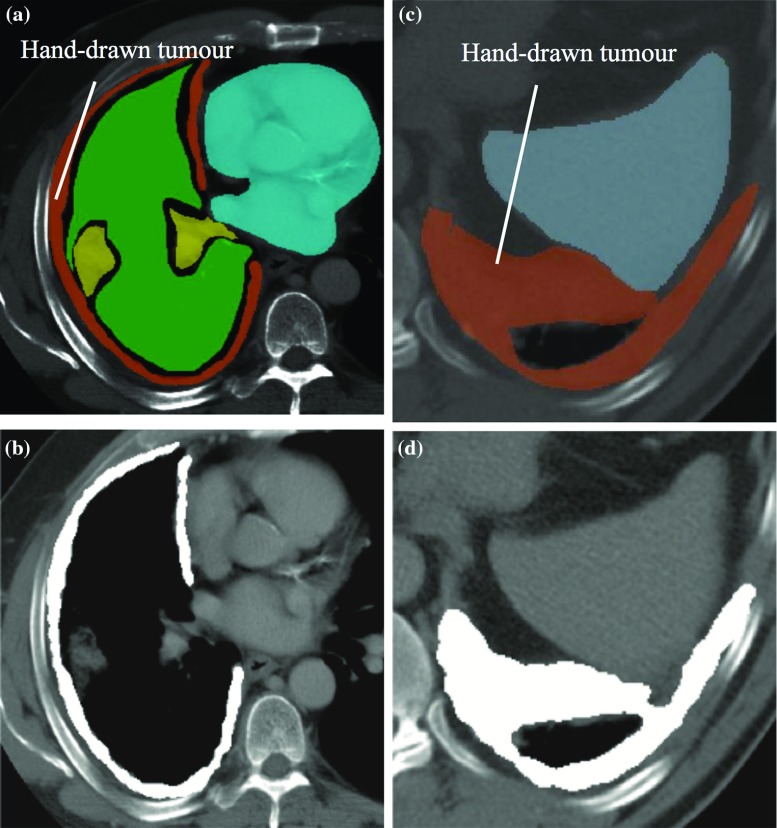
Segmented tumour contours on axial cuts of two arbitrarily selected CT scans, as shown in *white*. Manually delineated tumour contours are shown in *orange*. a, c Reference truth, b, d segmented tumour
